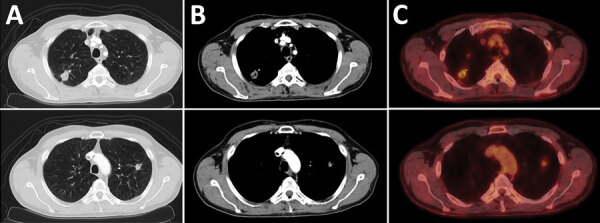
High-resolution computed tomography and 18F-fluorodeoxyglucose positron emission tomography scans of the chest showing pulmonary lesions caused by *Mycobacterium hassiacum* in a 62-year-old man, Austria. A and B) Computed tomography scans of the chest showing a subpleural thick-walled cavitary lesion in the posterior segment of the right upper lung lobe with associated pleural thickening and a smaller adjacent partly calcified solitary nodule. Another solid nodule of 13 mm diameter was found in the left upper lung lobe. C) Positron emission tomography scan showing a tracer uptake in both lesions with a standardized uptake values of 5 (top image) and 1.9 (bottom image).
